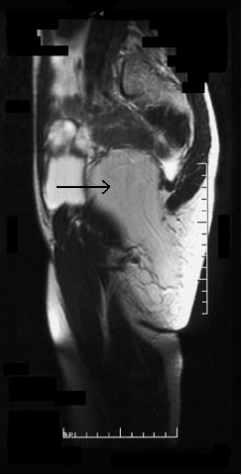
Sagittal T1-weighted MRI section (arrow indicating tumour).
